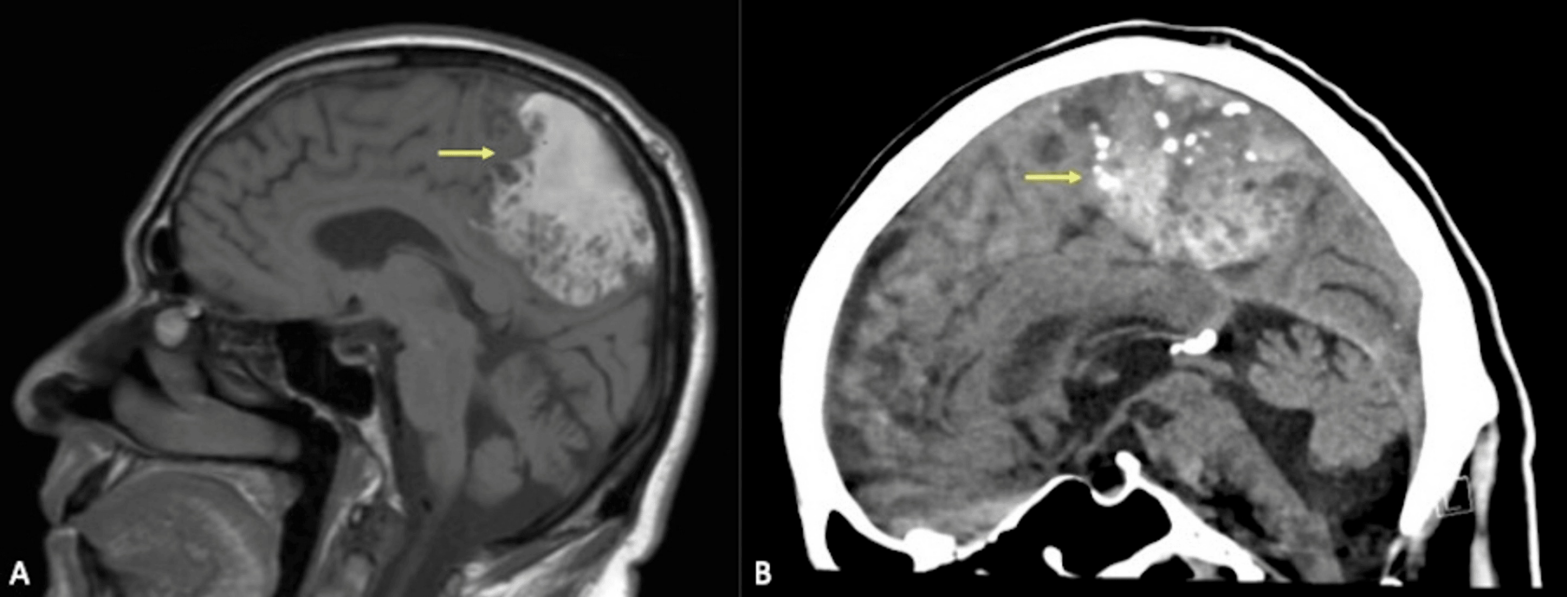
Sagittal CT and MRI sequences A: A sagittal non-contrast T1-weighted sequence depicts a markedly T1 hyperintense right frontoparietal lesion. B: A sagittal non-contrast CT scan again shows calcifications and hyperattenuation in the right frontoparietal lesion, corresponding to T1 hyperintensity on MRI and therefore indicative of intralesional high-protein content.
